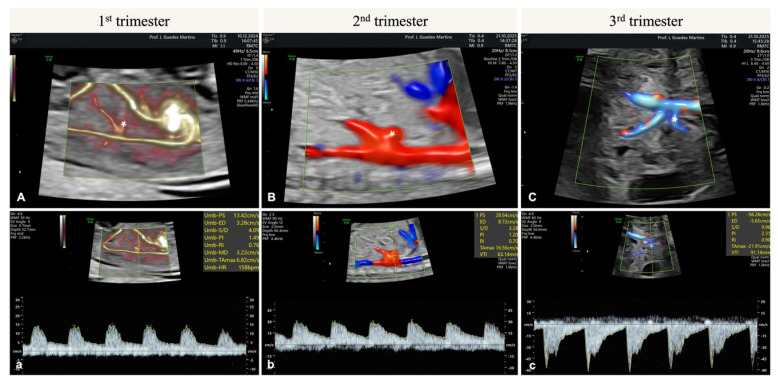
Fetal Hepatic Artery Doppler assessment during pregnancy. From left to right, the figure shows the Hepatic Artery (identified with a *) and its Doppler assessment showing normal velocity waveforms in the first (A,a), second (B,b) and third (C,c) trimesters. The HA (*) arises from the celiac artery—a branch of the abdominal aorta—and courses superoanteriorly towards the DV, with which it establishes close anatomical proximity. Throughout gestation, there is a decrease in both PI and RI, accompanied by an increase in PSV. This pattern reflects the physiological reduction in vascular resistance and increased blood flow to the fetal liver with progressive gestational age, paralleling the decrease in impedance observed in other fetal abdominal arteries throughout pregnancy.
